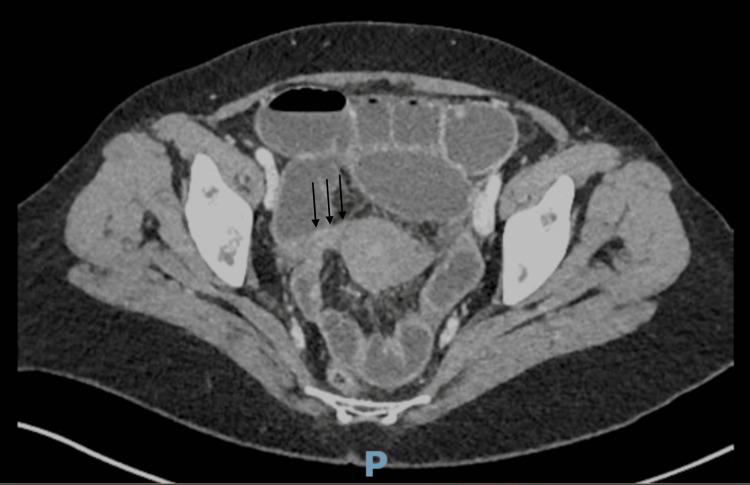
Axial slice of contrast-enhanced abdominopelvic CT showing small bowel distension with a pelvic transition zone suggestive of a broad ligament hernia or postoperative adhesion
